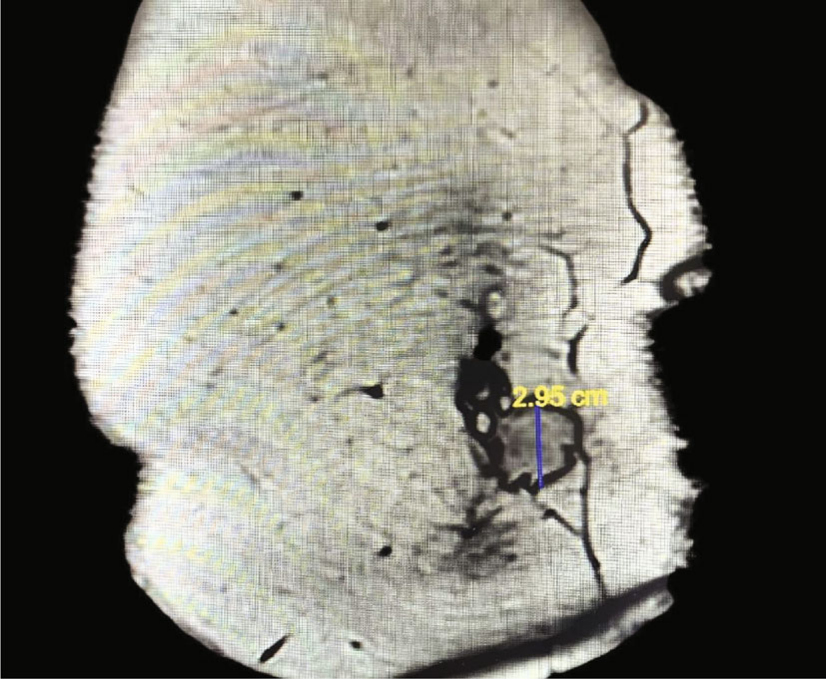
MRI measurement of the lesion.
